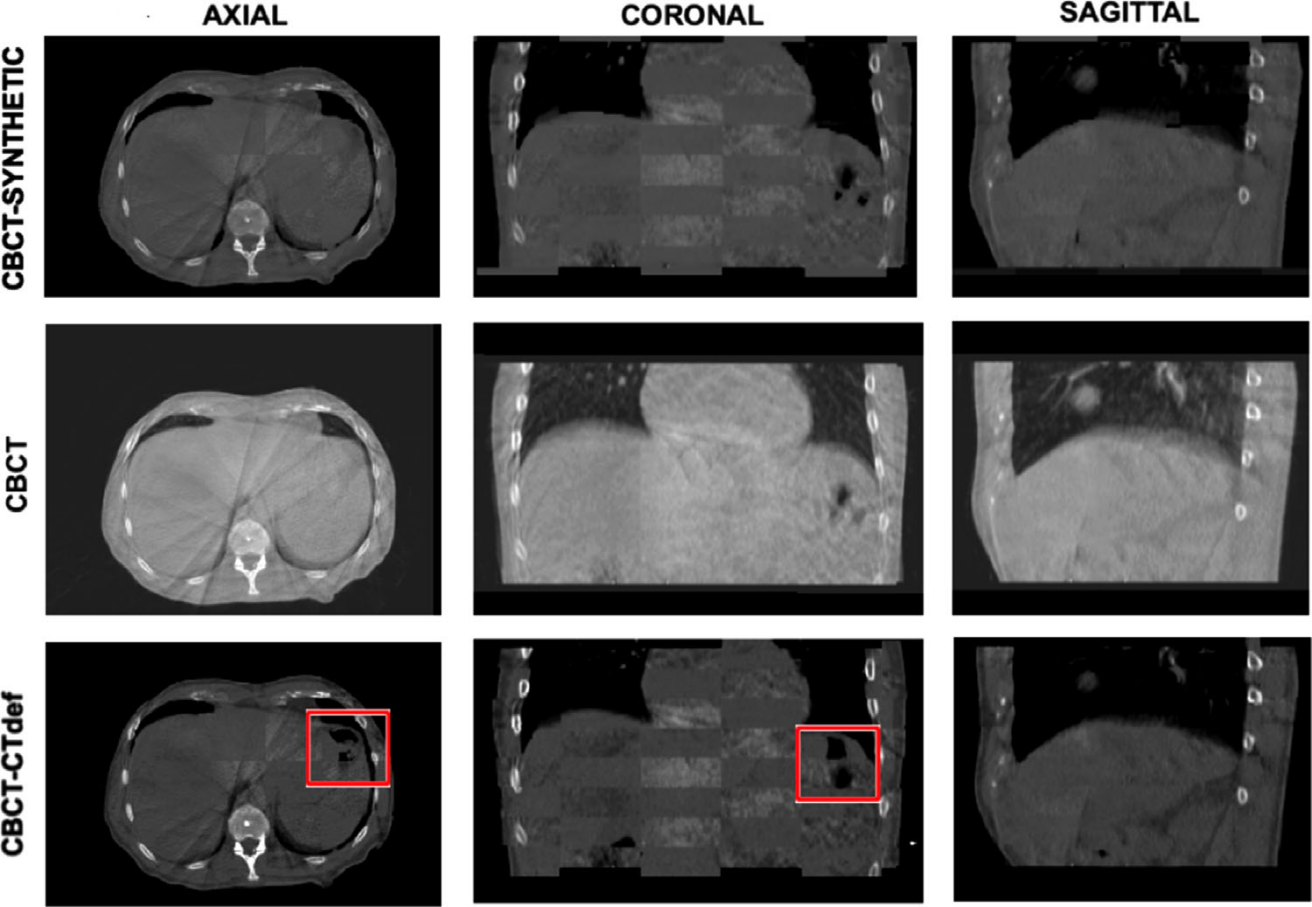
Anatomical preservation. Checkerboard pattern for the synthetic and cone beam computed tomography (CBCT) (top) image, CBCT image (middle), and checkerboard pattern for deformed CT (CTdef) and CBCT (bottom) images. Axial, coronal, and sagittal slices from left to right in that order. Window level and window width of the CBCT image was changed to W:1087 L: −88 with the purpose of better displaying the differences between the overlapped images.
